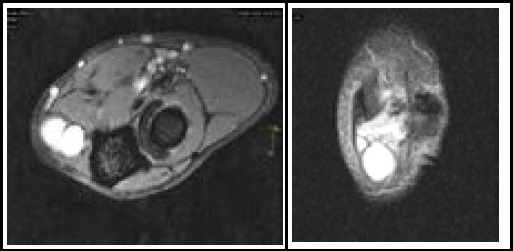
MRI of the left elbow joint showing a multiloculated cystic lesion in the posteromedial aspect with ulnar nerve stretched over its lateral border and degenerative changes. The contents of the lesion appearsT2/PD hyperintense.
